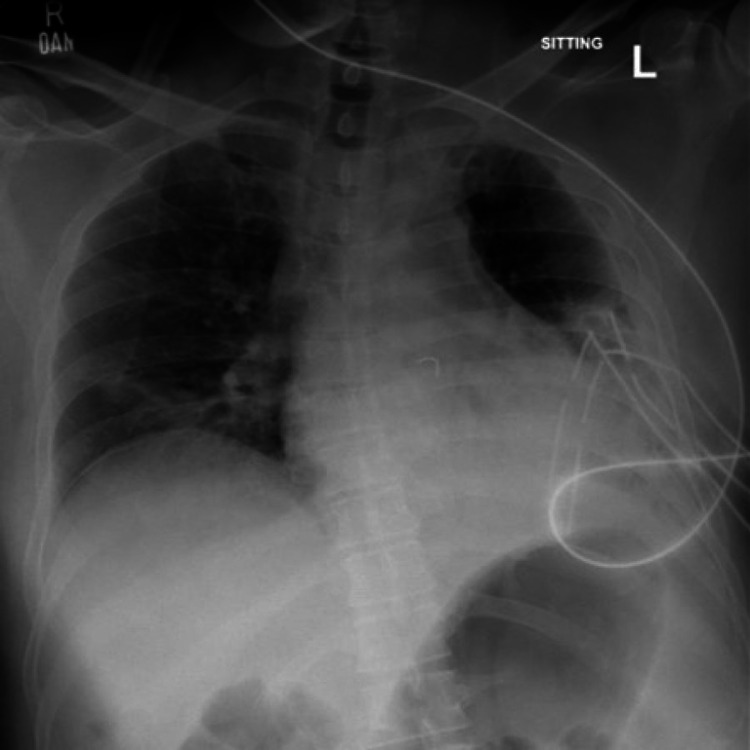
Post-VATS chest X-ray performed a year ago. Left-sided chest tubes are in place and empyema has resolved. The subtle radio-opaque density is shown by a black arrow. VATS: video-assisted thoracoscopic surgery
